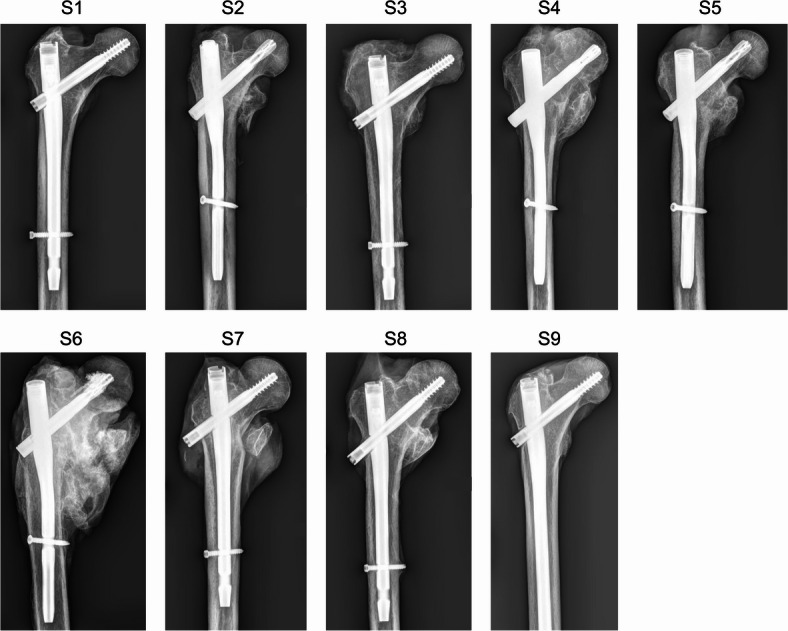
Anterior-posterior radiographs of the nine proximal femora used in this study.
